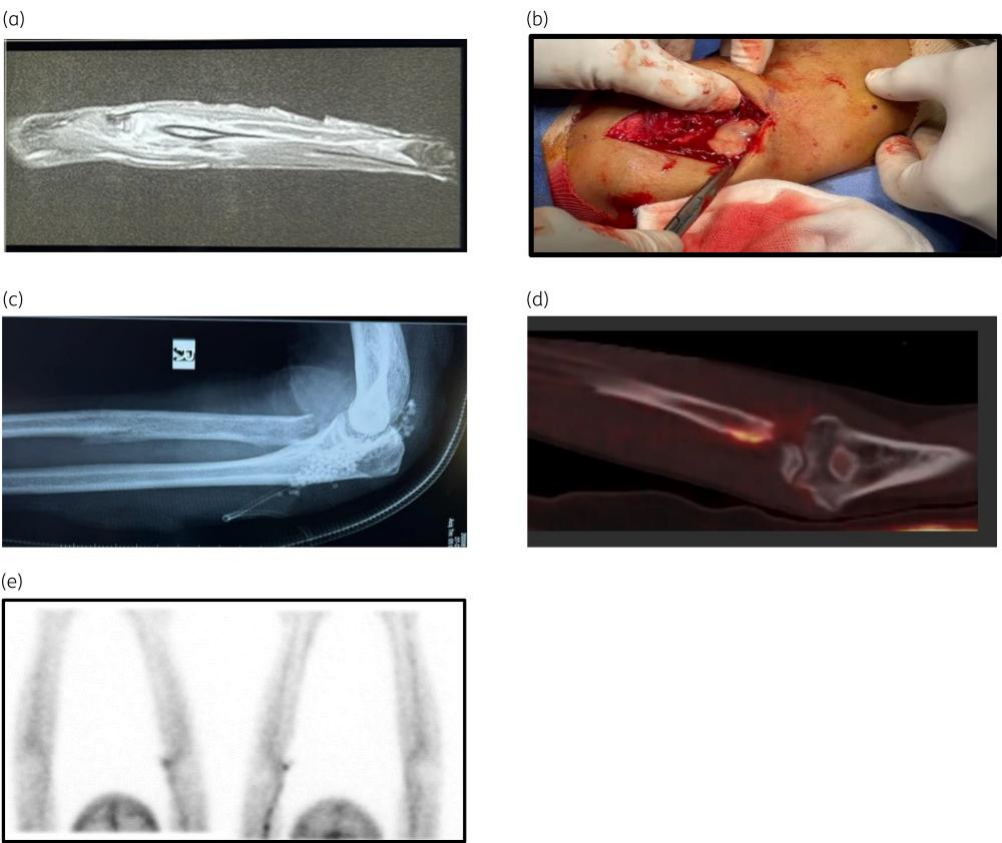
Images showing proximal radial osteomyelitis, surgical debridement, antibiotic stimulan placement and WBC scan. (a) Contrast MRI revealed proximal radial osteomyelitis with elbow joint effusion, (b) open debridement and radial head excision with frank pus coming out after the surgical incision, (c) antibiotic stimulan place, (d) ^18^F-FDG labelled autologous leucocyte PET CT scan, (e) bone scan with no uptake 3 weeks after discontinuation of the antibiotic.
